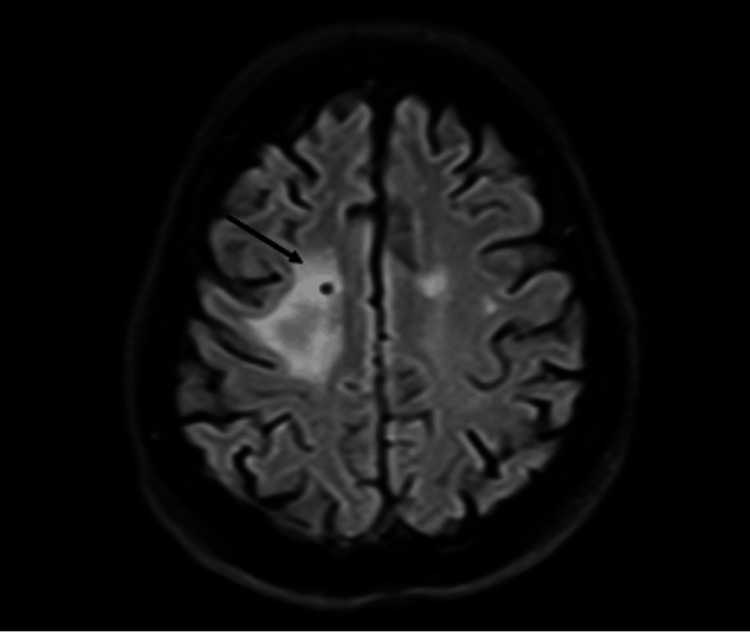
MRI from January 2024 showing findings consistent with a partial response of hemorrhagic supratentorial intra-axial metastatic disease
